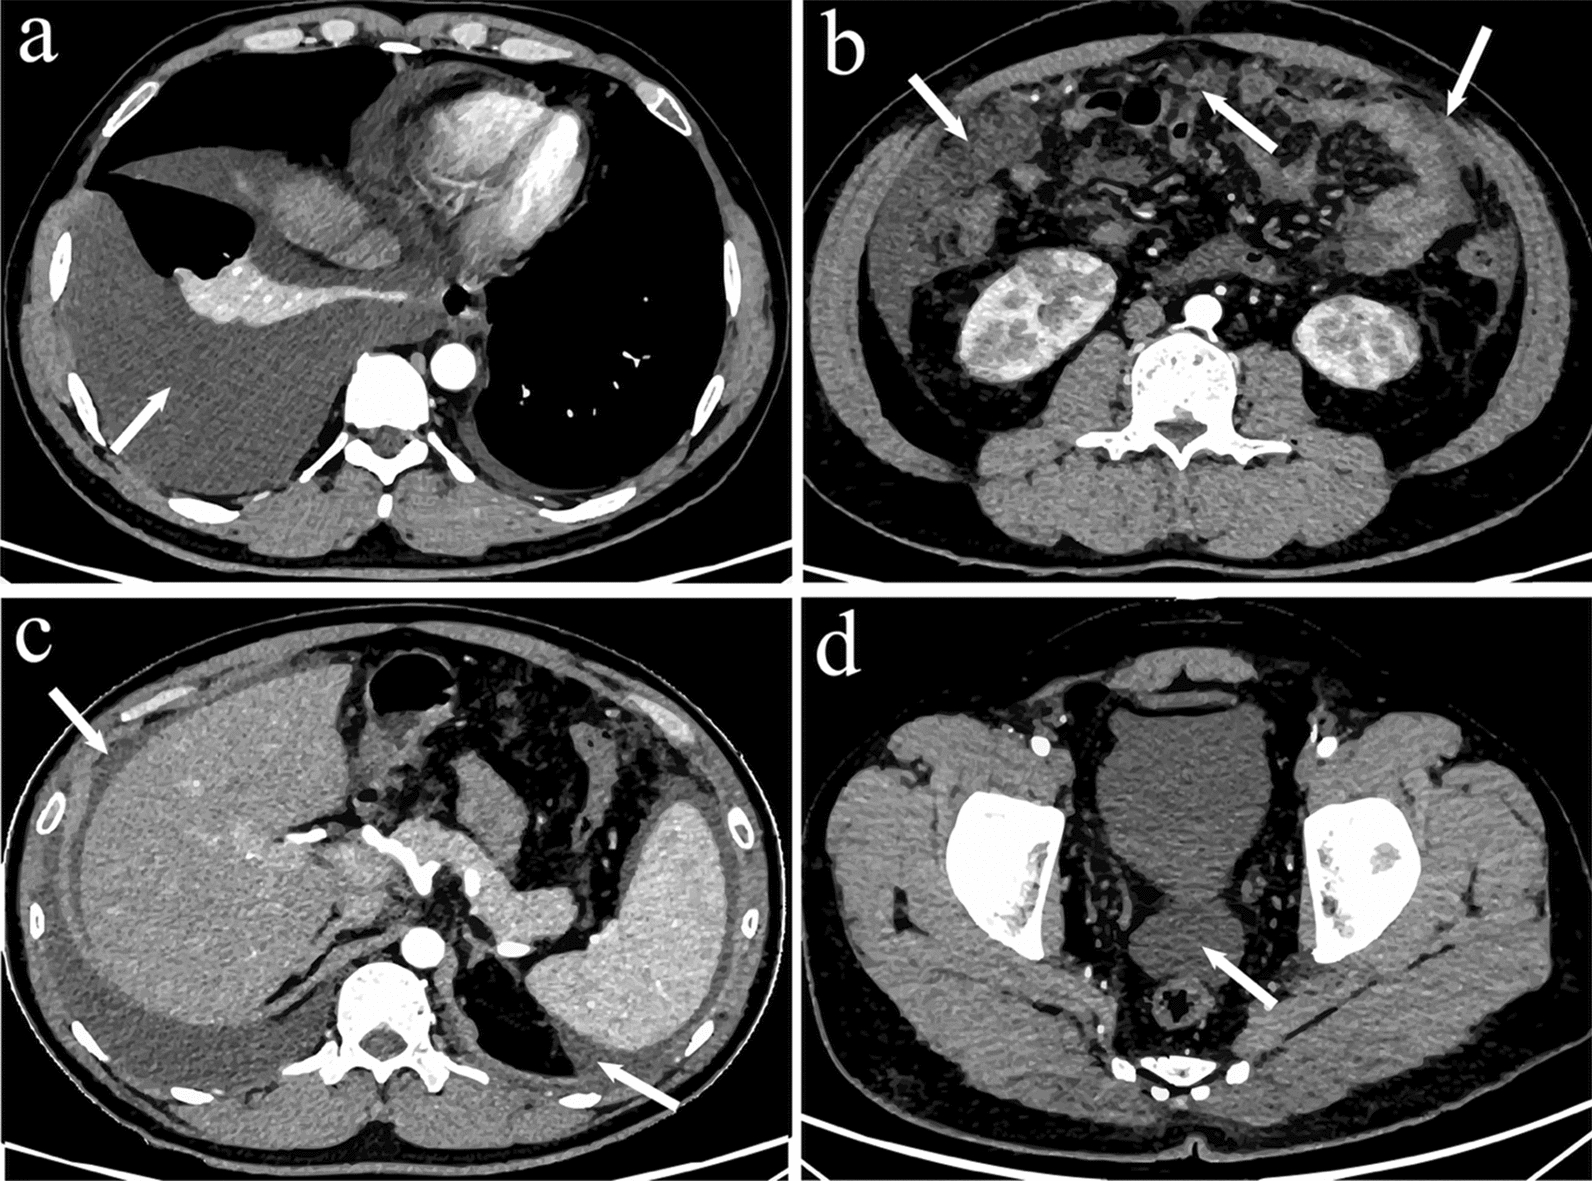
Contrast-enhanced Computed Tomography (CT) images demonstrating radiographic findings across different anatomical regions. a No abnormal parenchymal enhancement in the lungs. Bilateral pleural effusions (predominantly right‑sided) were accompanied by partial atelectasis of the right lower lobe. Minimal pericardial effusion (indicated by arrows). b Thickening of omental and mesenteric fat density, particularly in the omentum, displaying a "dirty" infiltrative appearance (indicated by arrows). c Fluid-density shadows along the hepatic and splenic surfaces (indicated by arrows). d Pelvic fluid collection (indicated by arrows)
